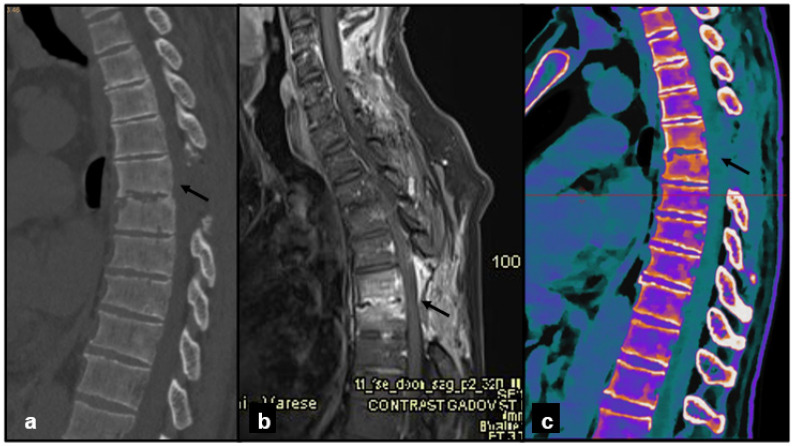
A 55-year-old male, work-related trauma. (a) Sagittal CT image, baseline acquisition of the thoracic spine shows intervertebral disc alteration with cortical irregularities of the opposing endplates (black arrow). (b) Sagittal MRI acquisition with PD/T2 FAT-SAT demonstrates intraspongious edema of the D5 and D6 vertebral bodies, indicating acute interstitial hemorrhage (black arrow). (c) Sagittal DL-SCT image, post-processed with IntelliSpace system, highlights the presence of edema through increased electron density, visualized in orange coloring (black arrow). Color bar legend. White: normal cortical bone; orange: intermediate to high ED; purple: low ED; blue: very low ED, consistent with normal bone marrow composition rich in fat.
